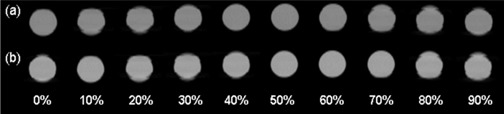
Representative images of 4D ${\text{CT}}_{\text{SBV}}$ (a) and 4D ${\text{CT}}_{\text{RPM}}$ (b) of the imaging object.
